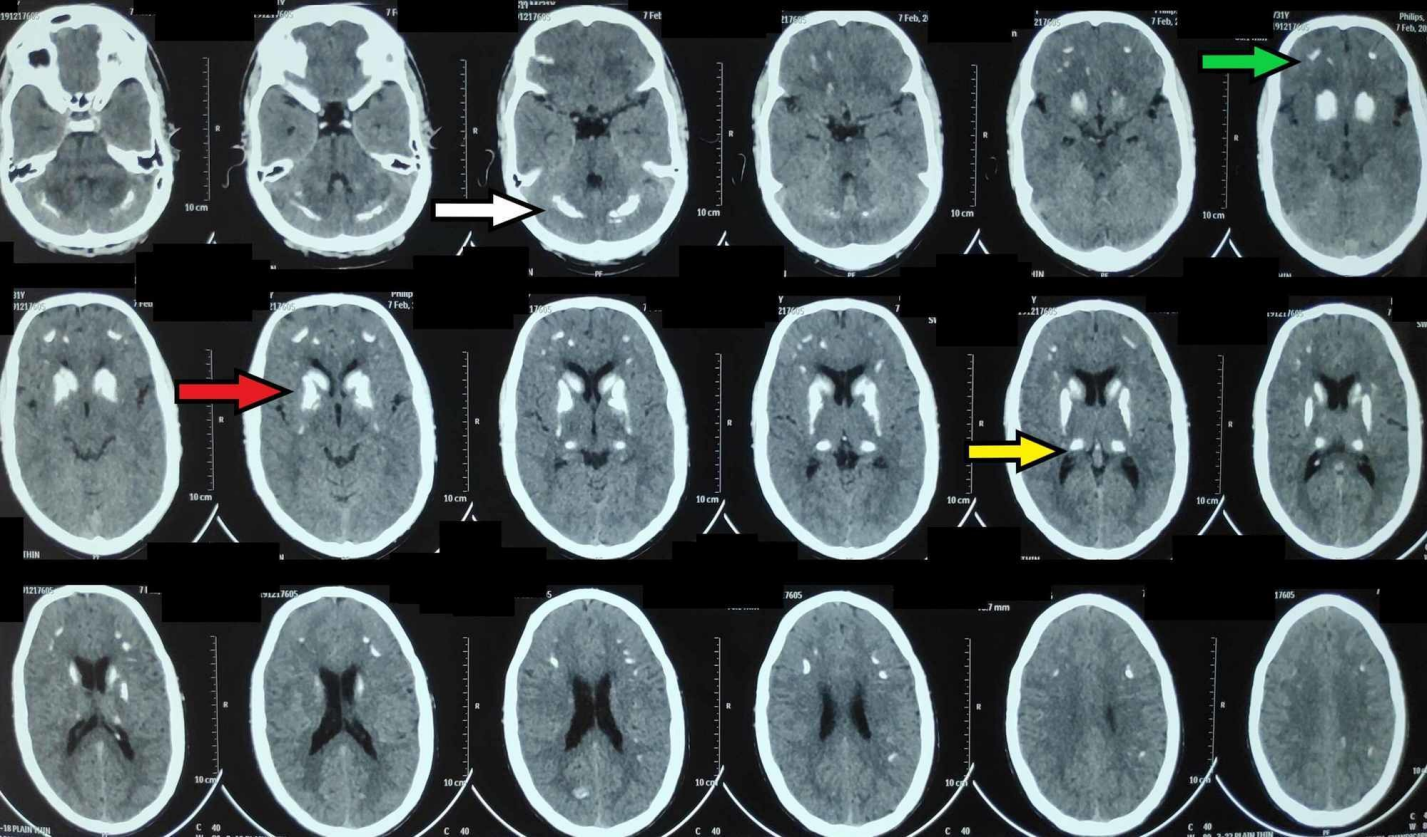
Axial sections of a CT scan of the brain showing bilateral symmetrical calcification CT, computed tomography The calcification is evident as hyperdensity, predominantly in bilateral basal ganglia (red arrow) and thalami (yellow arrow). Smaller lesions are also seen in the frontal cortex (green arrow) and cerebellum (white arrow).
